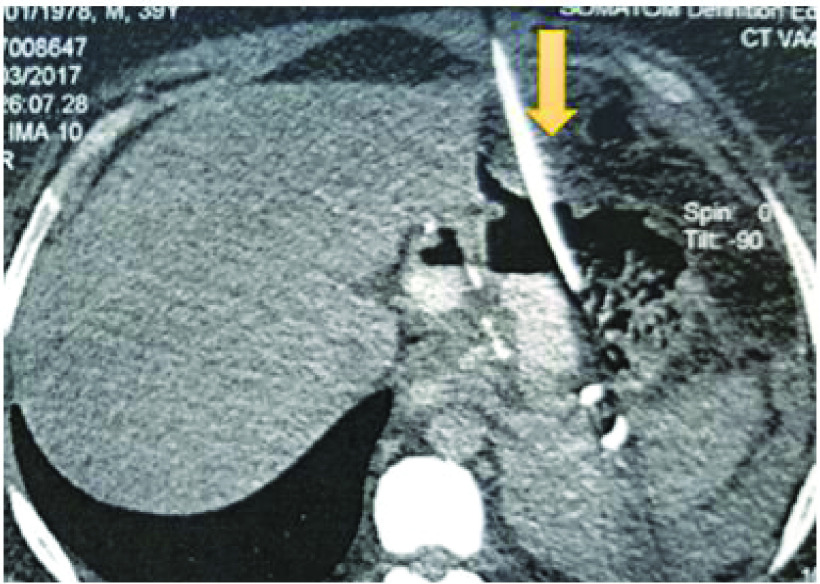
A coronal abdominal CT section showing the percutaneous radiological drainage (yellow arrow).
